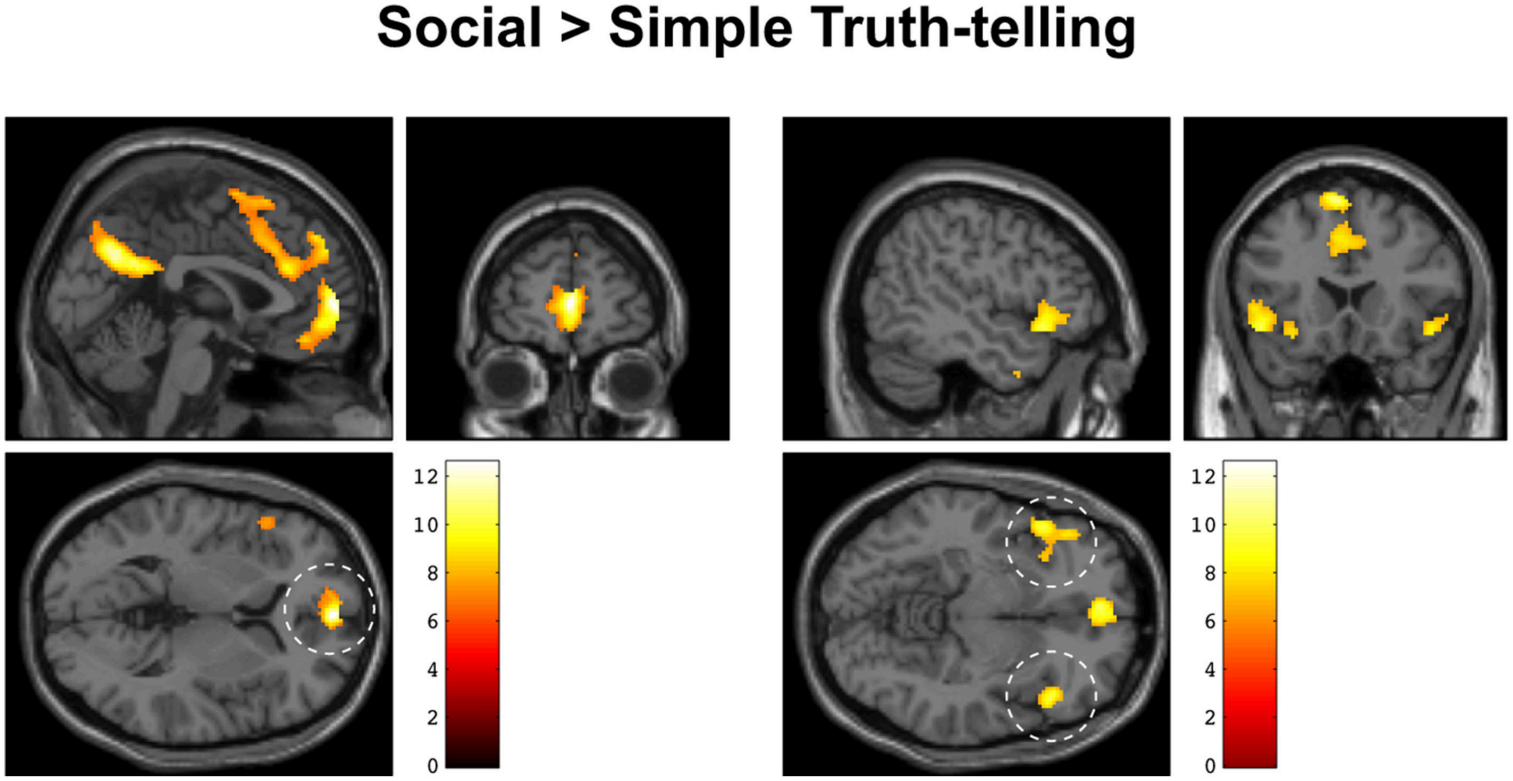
Increased BOLD activity during social > simple truth telling overlaid on an anatomical MRI of a single subject in MNI space. Left: sagittal, coronal, and axial sections centered on the medial prefrontal activation (dashed circle). Right: sagittal, coronal, and axial sections centered on the activation in bilateral anterior insula and inferior frontal gyrus (dashed circles).
